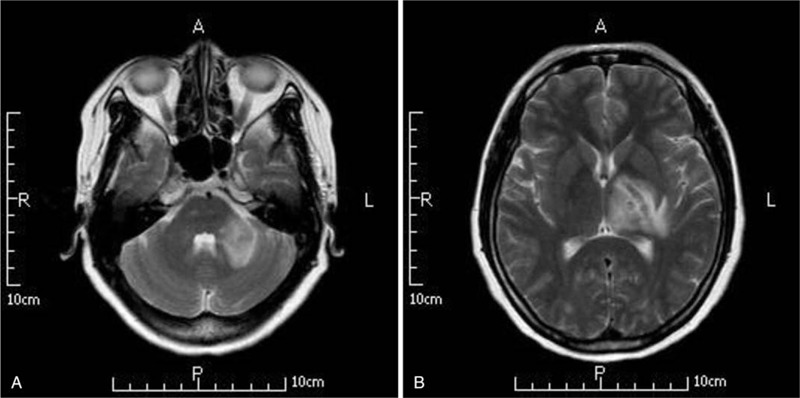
(A and B) The head MRI revealed some new lesion compatible with tuberculoma after a standard 3 mo care with anti-tuberculosis drugs and corticosteroids. MRI = magnetic resonance imaging.
